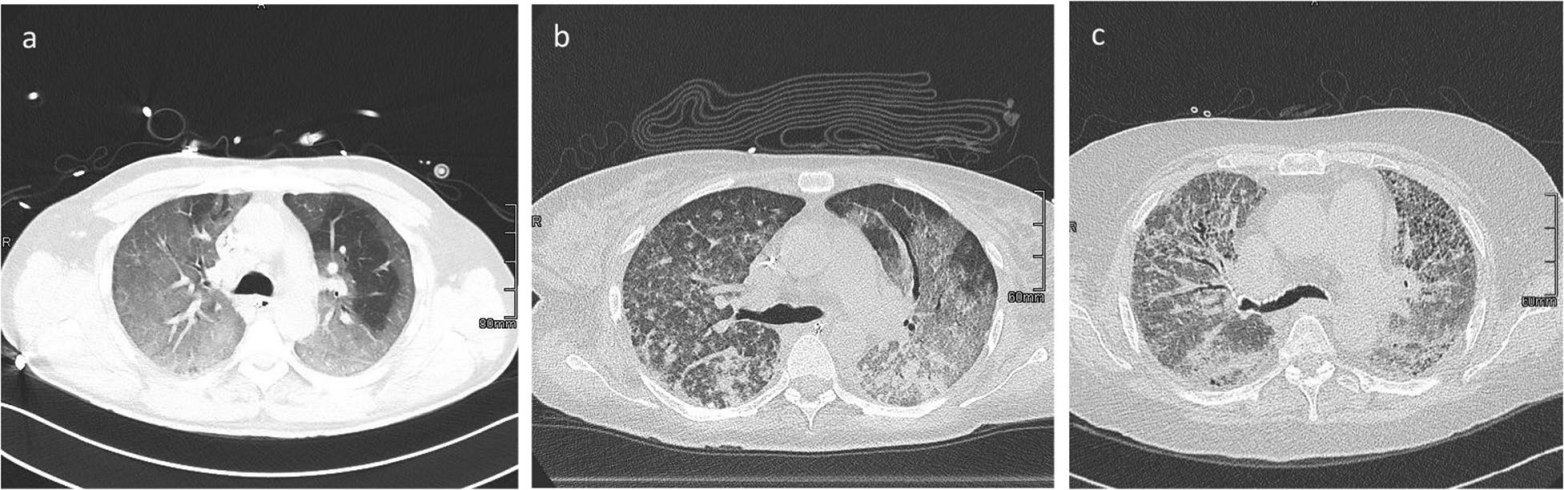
Examples of CT coarseness sore. a Coarseness score 1 (fine intralobular fibrosis); b coarseness score 2 (microcystic reticular pattern comprising air spaces smaller than or equal to 4 mm in diameter); c coarseness score 3 (macrocystic reticular pattern comprising air spaces larger than 4 mm in diameter)
